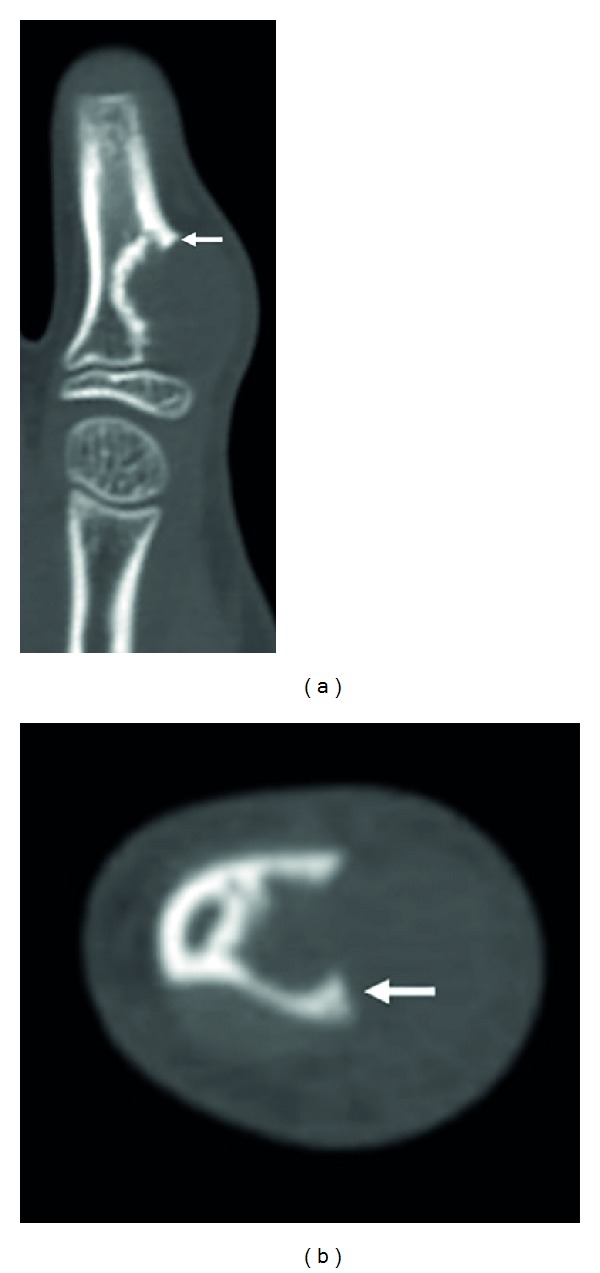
Coronal (a) and axial (b) computed tomography scans show cortical overhanging margins at the periphery of the lesion (arrows).
